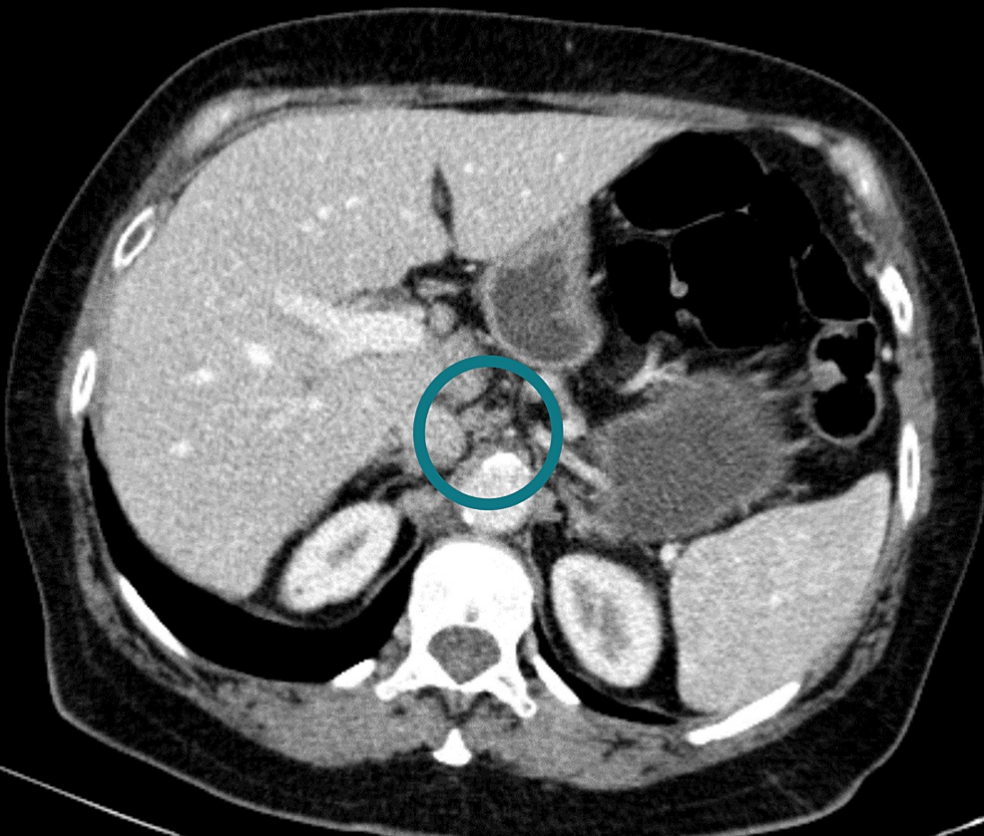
Abdominal CT from 2020, after treatment with ursodeoxycholic acid and without enlargement of ganglia.
